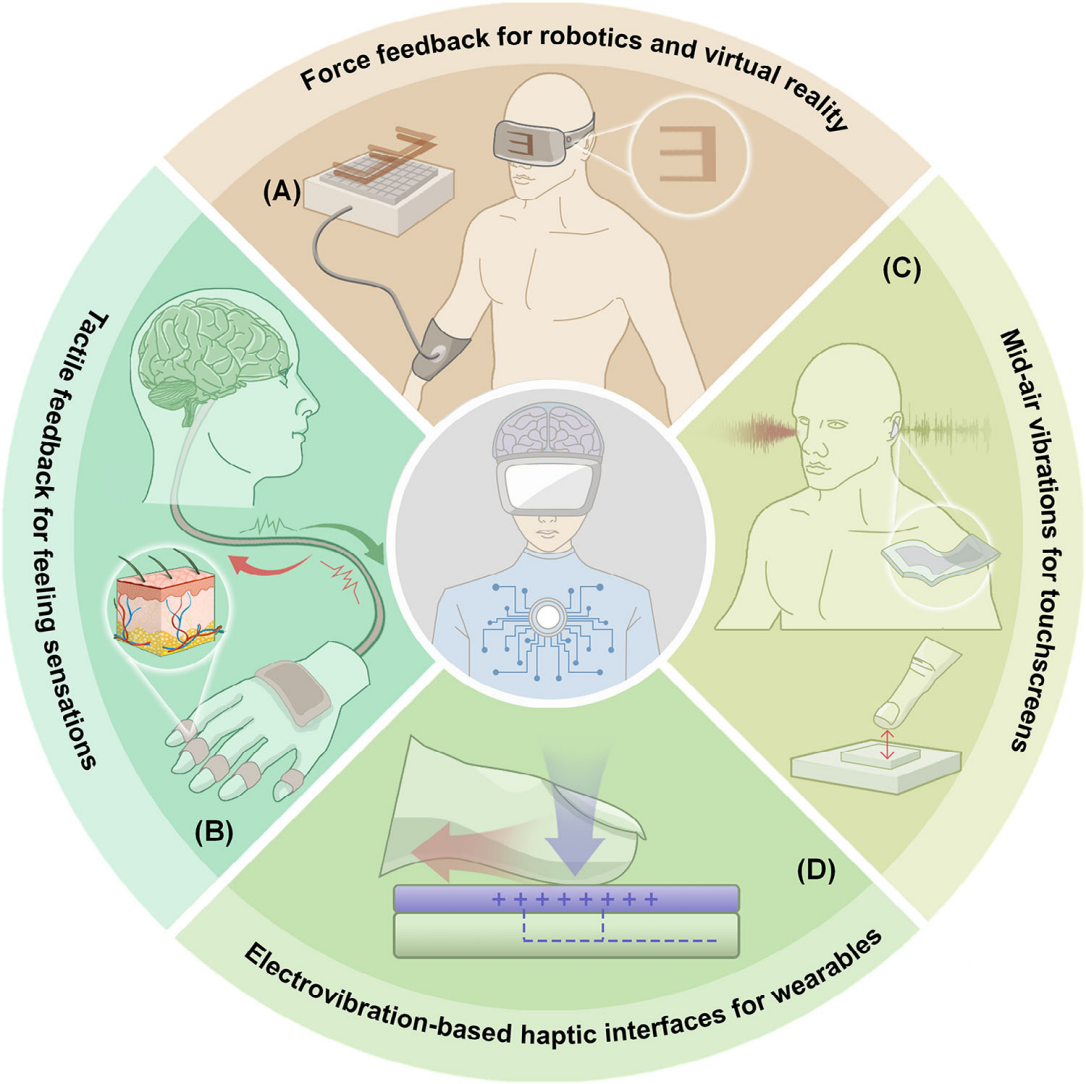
Neural interfaces for haptic interactions. (A) Force feedback for robotics and virtual reality. (B) Tactile feedback technologies, such as triboelectric sensors, designed for feeling sensations. (C) Mid‐air vibrations for touchscreens and similar interfaces, incorporating technologies like ferroelectric, electromagnetic, ultrasonic piezoelectric, and thermoelastic laser devices. (D) Electrovibration‐based haptic interfaces for wearables such as gloves, featuring pneumatic, piezoelectric, thermoelectric, or ferroelectric actuators; and heating haptic interfaces.
